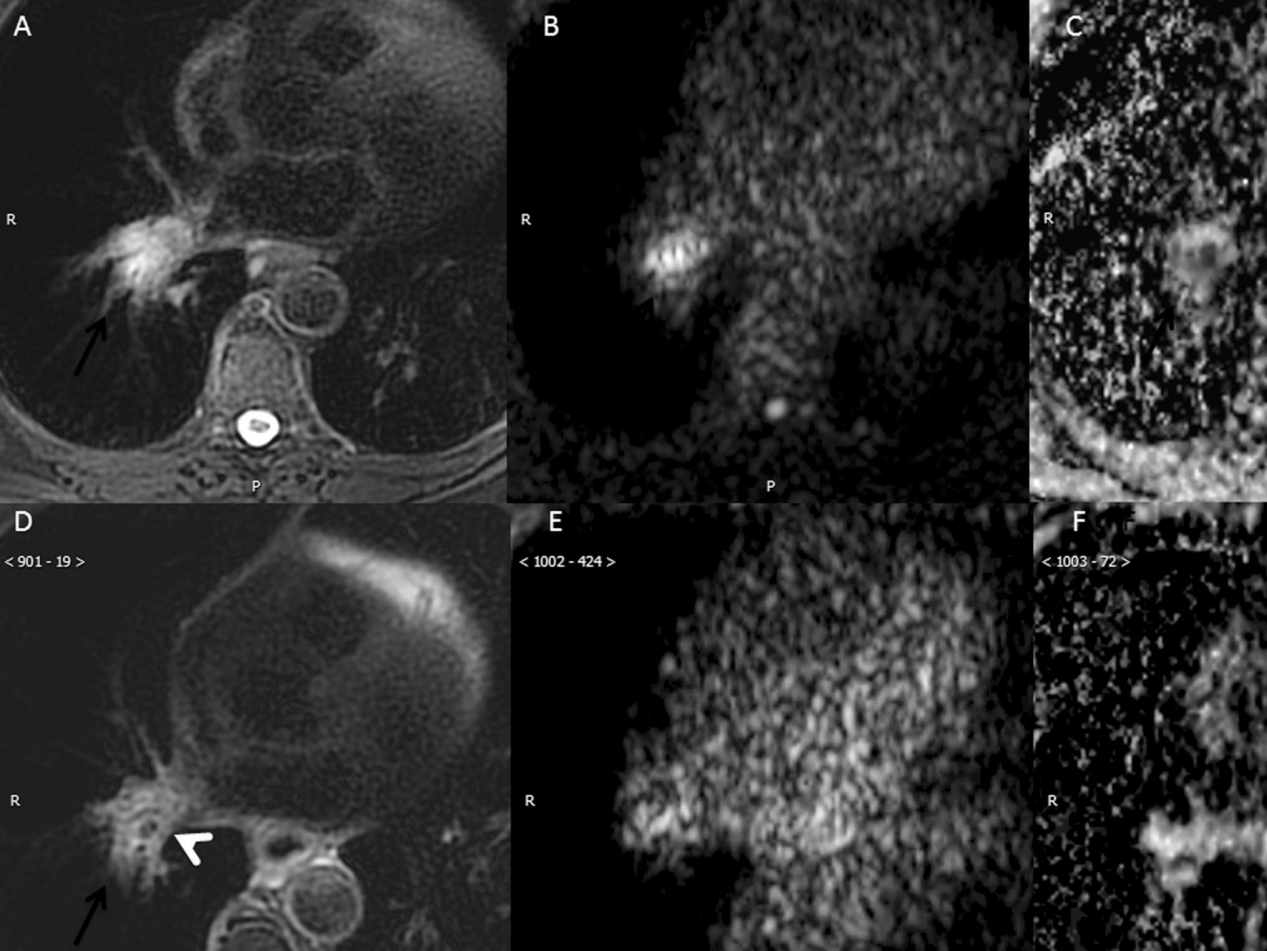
Follow-up MRI studies in a 62 year-old male with lung invasive adenocarcinoma stage IIIA (arrows) treated (a–c) Pretreatment MRI (top row images) shows a primary right hilar mass of 29 mm invading main and intermediate bronchi, which shows high signal intensity on fat-suppressed T2-weighted image (a) and high signal on high b value DWI (b) and low signal on ADC map (ADC: 1.18 × 10^−3^ mm^2/^s) (c), representing true restriction diffusion and related to the high aggressiveness of the mass. After 2 months of treatment neoadjuvant chemotherapy, a new MRI was performed to evaluate surgical options (bottom row images), demonstrating a significant reduction of the right hilar mass (maximum diameter of 19 mm) and with recanalization of the intermediate bronchus on fat-suppressed T2-weighted image (d). In addition, there is a reduction of the signal intensity of the lesion on high b value image (e) and increase of ADC value (1.58 × 10^−3^ mm^2^/s) representing good response to treatment. This case shows how DWI can help in treatment selection as a pretreatment low ADC value has been related to good response to chemotherapy
